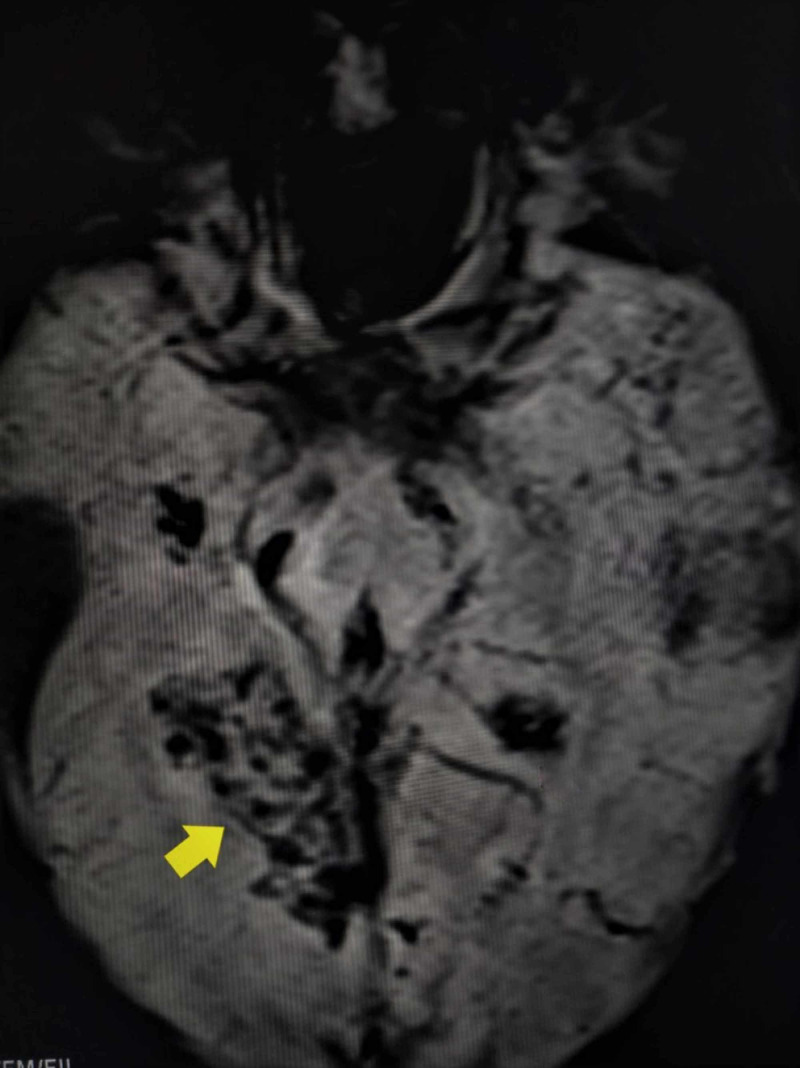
GRE sequence of CEMRI The image shows a blooming (yellow arrow) pattern CEMRI: contrast-enhanced magnetic resonance imaging; GRE: gradient echo
